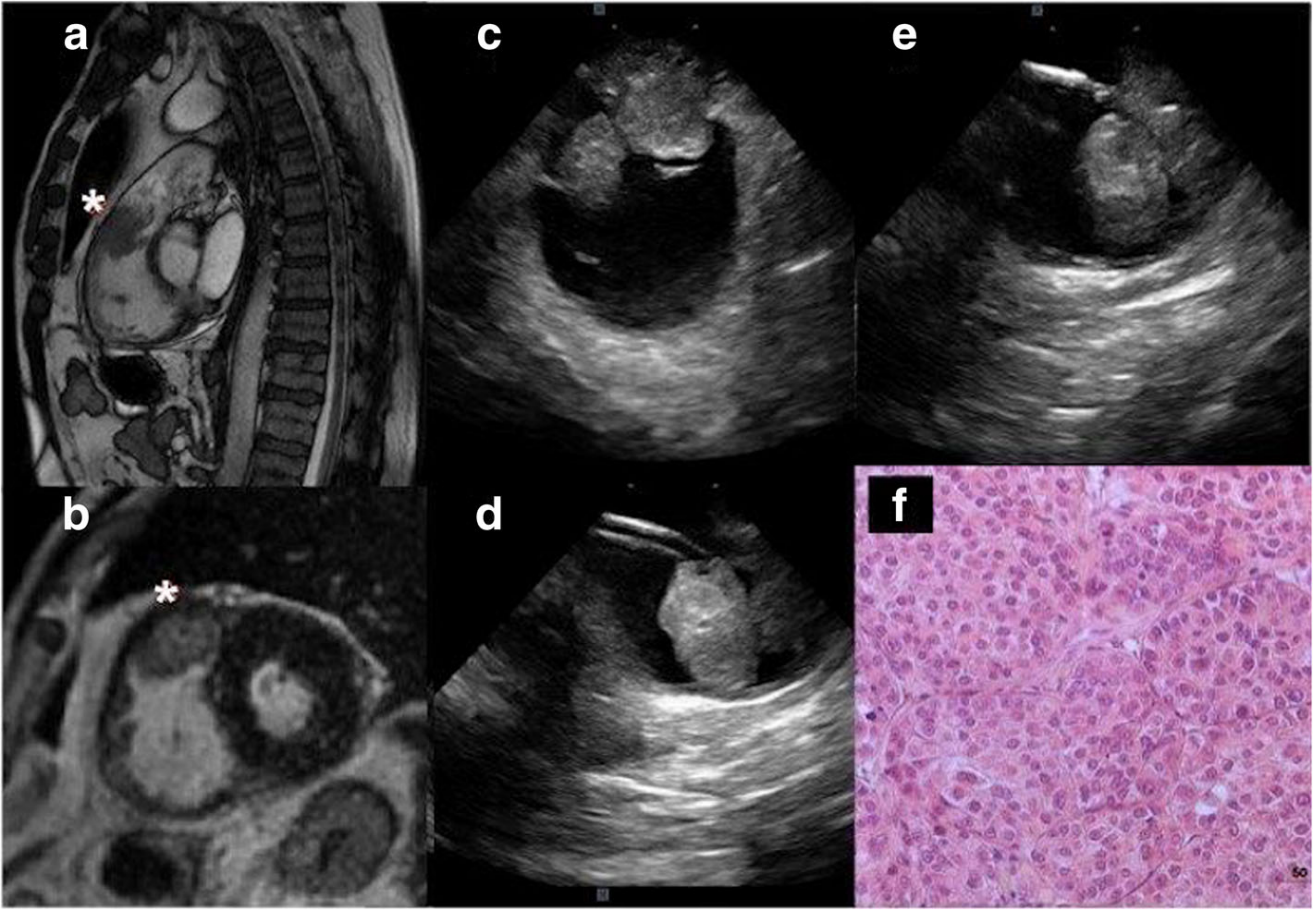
Cardiac magnetic resonance imaging: a rounded 30 × 17 mm mass based in the anterior portion of the right ventricular outflow tract (RVOT) involving the pulmonary valve is showed in panel (a and b) (white star). ICE clearly shows the mass, panel (c), and is useful to guide the correct positioning of the long-deflectable sheath through which the bioptome is introduced, panel (d). ICE visualizes a good contact between the bioptome and the mass, panel (e). Hystopathological diagnosis revealed a metastatic localization of hepatocellular carcinoma, panel (f)
